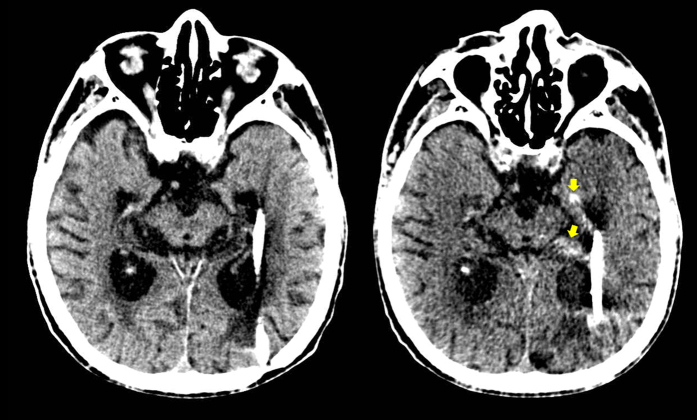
Confirmation of the direct CSF communication between the lateral ventricles and the ambient cistern by shuntography in patients with iNPH with a suspected shunt malfunction. Contrast medium (yellow arrow head) was observed not only in the left inferior horn of the lateral ventricles but also in the left ambient cistern on CT scan immediately after shuntography (right), compared to that before shuntography (left).
